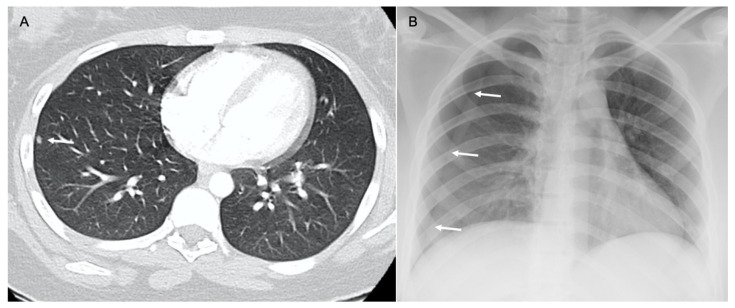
Patient with osteosarcoma and lung metastasis presented with spontaneous pneumothorax. Axial enhanced CT image (A) shows a right lower lobe subpleural lung nodule (arrow). Frontal chest radiograph (B) 5 days after the CT shows a medium-size right pneumothorax (arrows).
